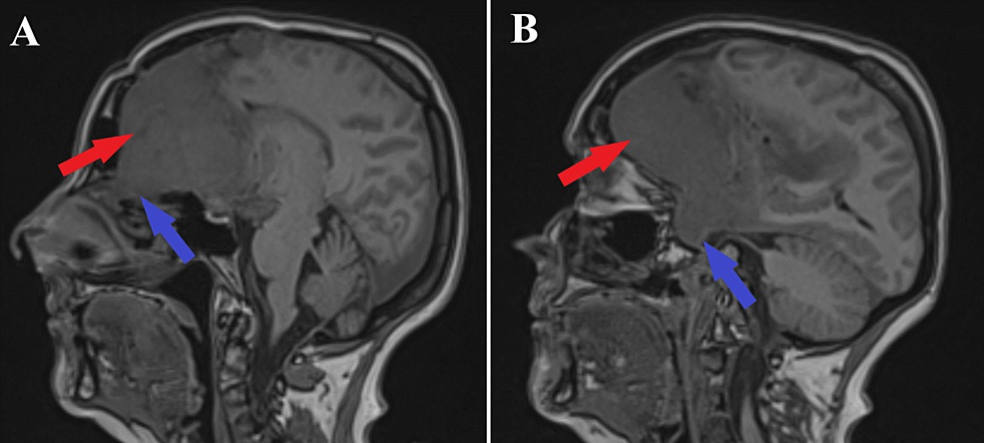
Preoperative MRI T1-sequence, sagittal section. Both images depict the tumoral process (indicated by red arrows) with significant orbital extension (A, shown by the blue arrow) and pituitary stalk implication (B, highlighted by the blue arrow).
